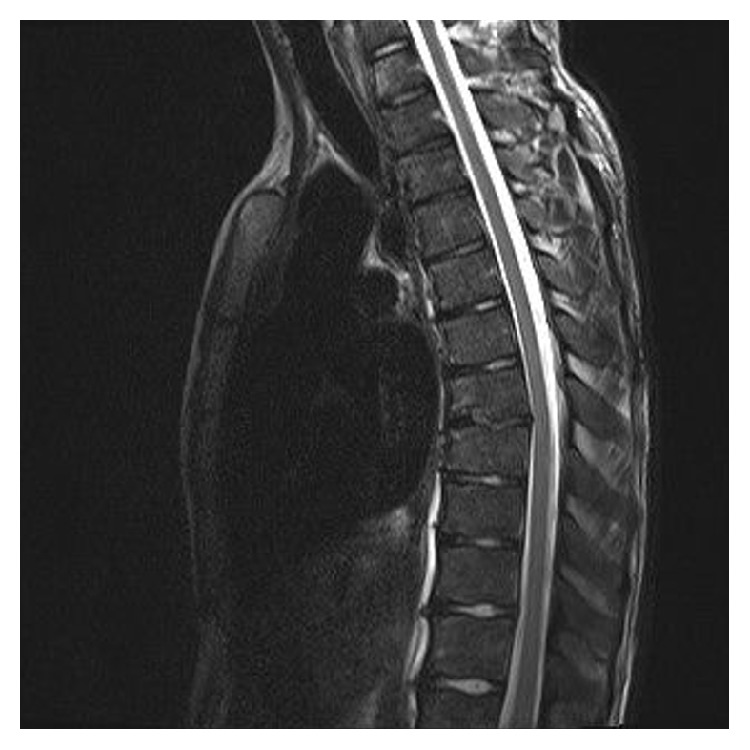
MRI of the spine showing the fracture of the base plate in thoracic spine body 7.
